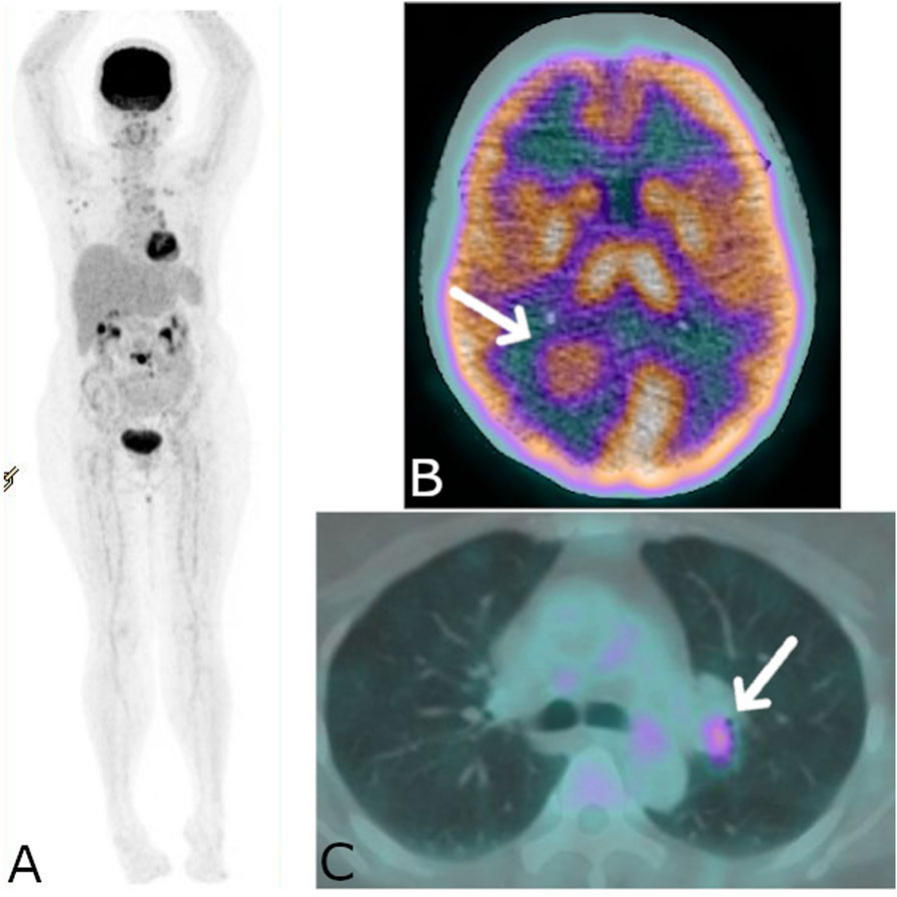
FDG PET/CT of patient number 2. a MIP showed multiple hypermetabolic lesions. b hypermetabolic nodule at brain highly suspicious for metastasis. c hypermetabolic lesion at left hilar region
